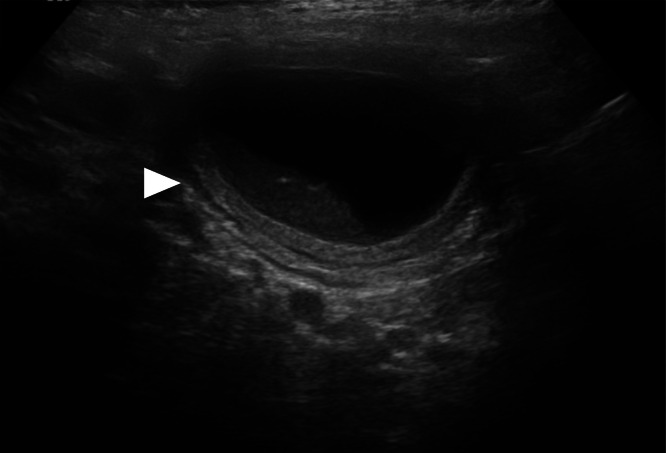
Longitudinal ultrasound image showing an oval, anechoic cystic lesion with well-defined borders and a hyperechoic “*double-wall”* appearance (white arrowhead). Internal fine echogenic debris is present in the dependent portion, and no vascularity is seen within the lesion on Doppler examination. The cyst is situated medial to the cecal wall.
